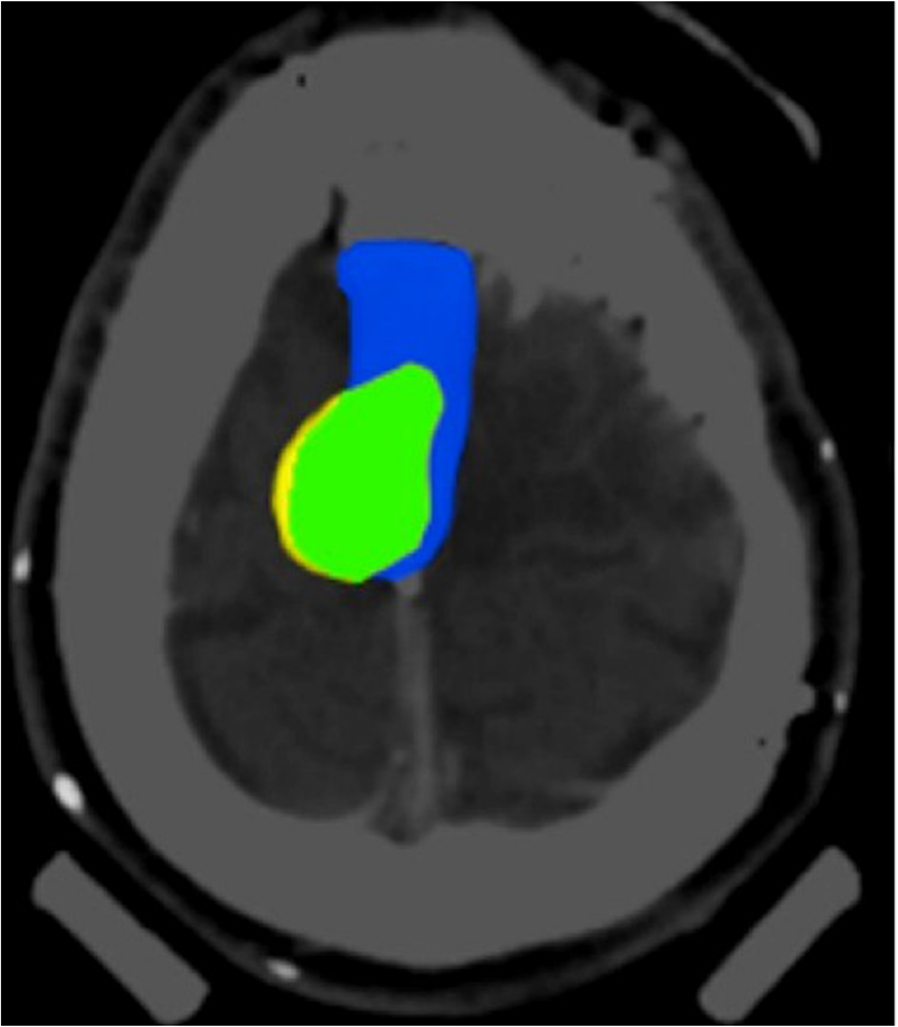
Corresponding volume (green) of GTV_CT/MRI_ (blue) and GTV_FET_ (yellow) of one patient. For more details of this patient including CT-, MRI-, DOTATOC- and FET-PET-images see Fig. 5a
